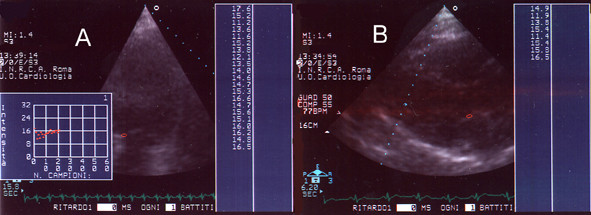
Score 1. Panel A: AD of mitral annulus (apical scan); Panel B: AD of aortic valve (parasternal scan).
